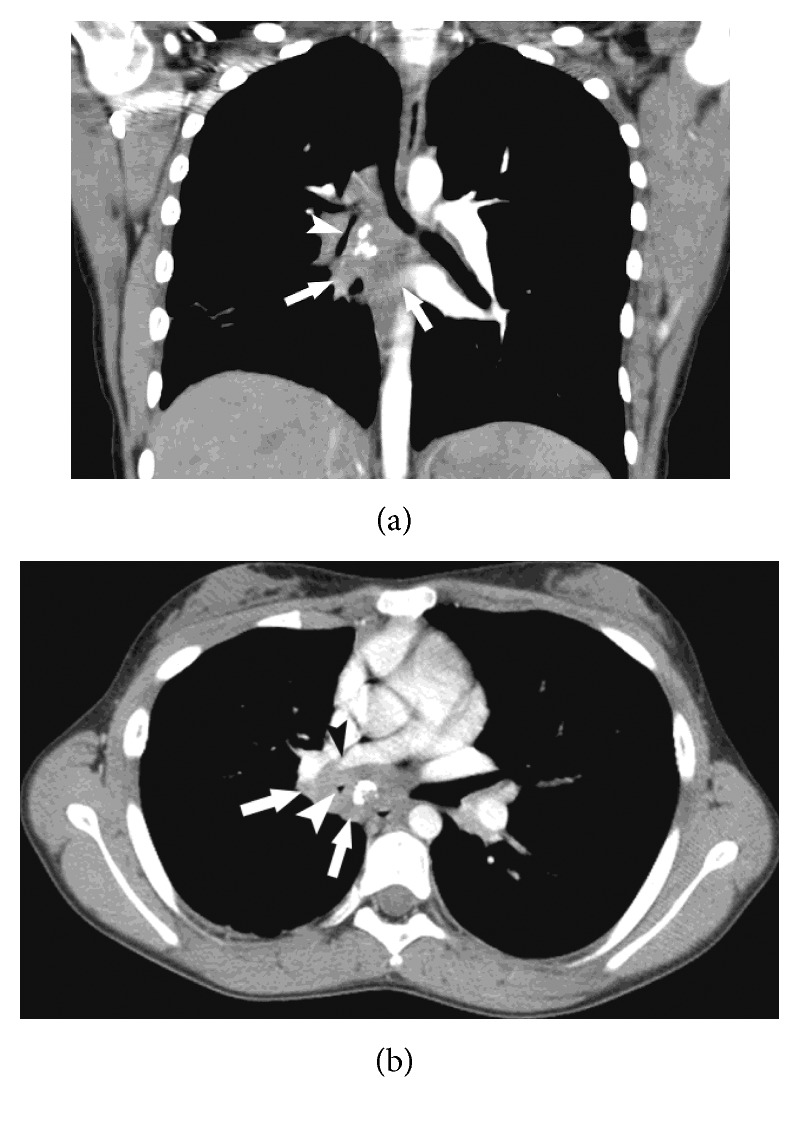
Axial (a) and coronal (b) reformats of the chest CT following a contrast injection, showing a subcarinal and right hilar ill-defined soft tissue mass (white arrows) with prominent interior calcifications causing marked compression on the bronchus intermedius, right middle lobe bronchus (white arrowhead), and right inferior pulmonary vein (black arrowhead).
